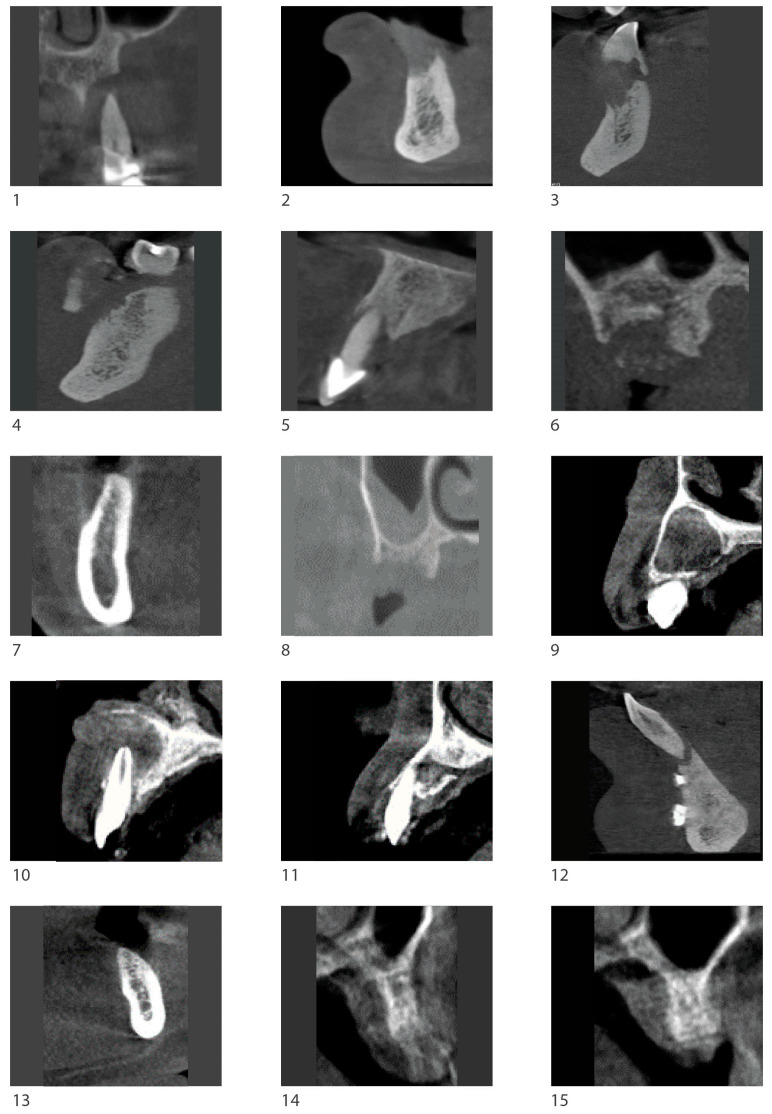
Sagittal pre-surgical CBCT images showing the residual atrophic area before surgery. Numbers 1–15 represent the 15 cases described, nested within 11 patients. Using the tooth numbering system for identification, the location of each case is described as follows: Case 1: Tooth 25, Case 2: Tooth 42, Case 3: Tooth 33, Case 4: Tooth 34, Case 5: Tooth 21, Case 6: Tooth 17, Case 7: Tooth 46, Case 8: Tooth 26, Case 9: Tooth 13, Case 10: Tooth 11, Case 11: Tooth 13, Case 12: Tooth 41, Case 13: Tooth 36, Case 14: Tooth 25, Case 15: Tooth 26.
